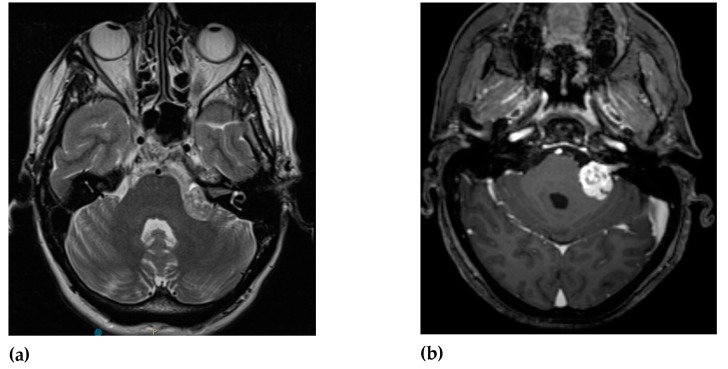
MRI imaging of sporadic vestibular schwannoma (VS) in cerebellopontine angle: (a) T2-weighted MRI reveals sporadic VS as hypointense signals and (b) T1-weighted image with contrast demonstrates hyperintensity of sporadic VS (courtesy of Y. Temel).
